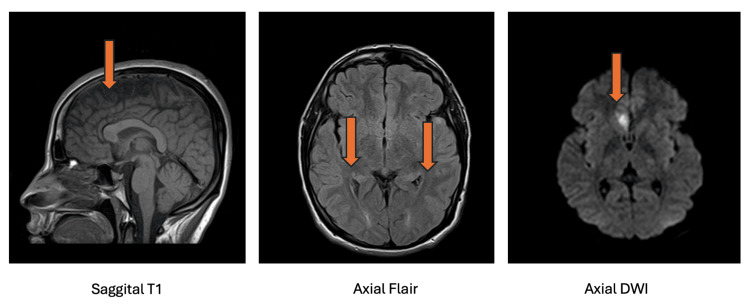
Areas of high FLAIR signal with restricted diffusion on axial DWI are observed in the corpus callosum (genu and splenium), the subcortical white matter of the right temporal lobe, the left occipital region, and the posterior parasagittal parietal regions, consistent with DAI grade II (arrows). FLAIR: Fluid-Attenuated Inversion Recovery; DWI: diffusion-weighted imaging; DAI: diffuse axonal injury The images are taken from Haouimi (2025) [58]. This content is covered under CC BY-NC-SA 3.0.
